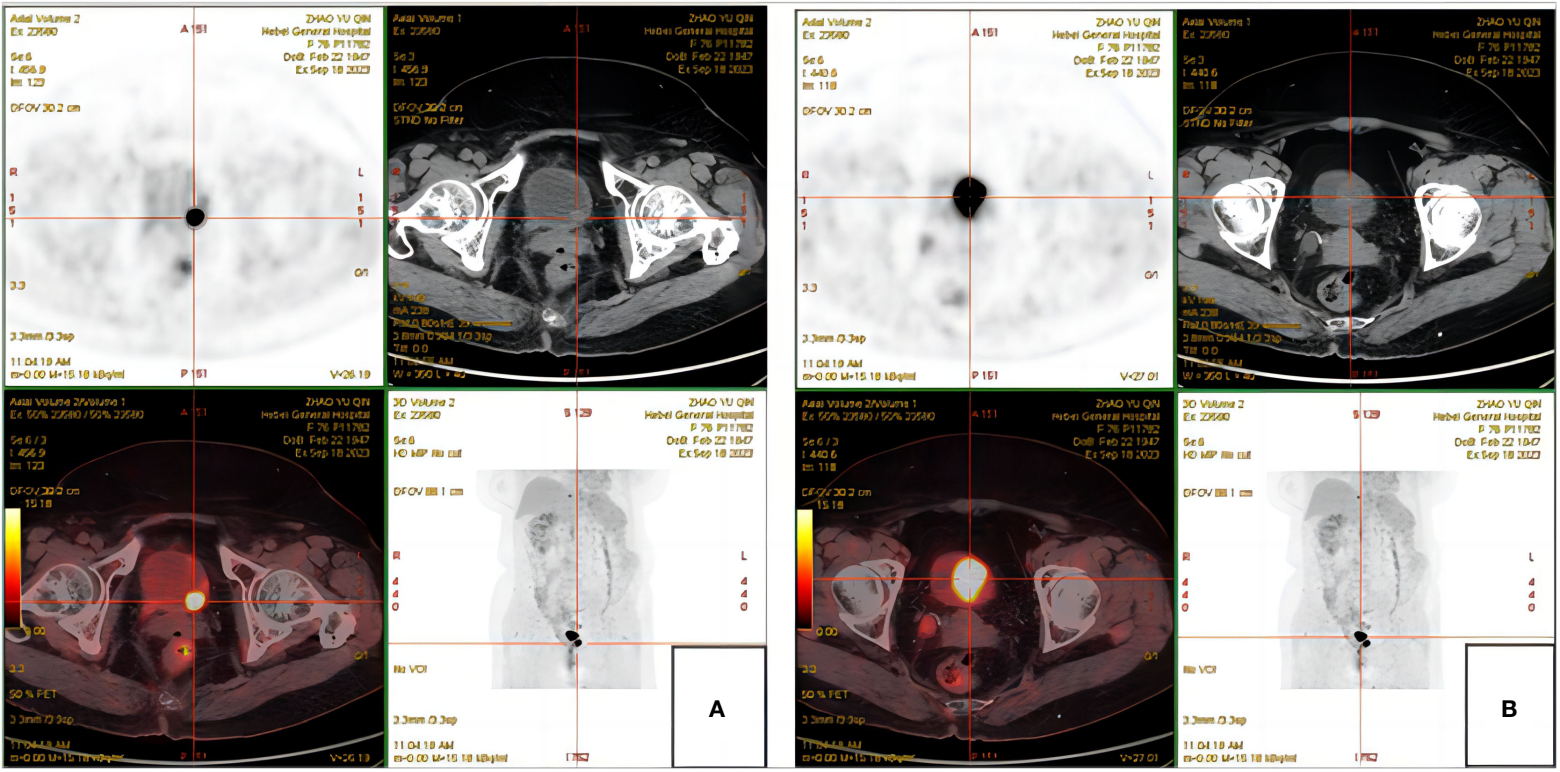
PET-CT. (A) High metabolic wall occupation in the left posterior wall of the bladder. (B) High metabolic wall occupation near the upper margin of the bladder body.
